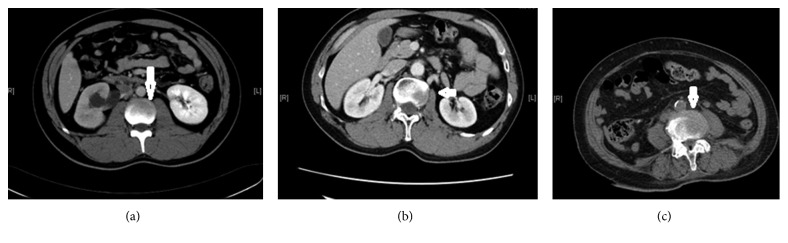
Tumor spread to the vertebral body. CT scans showing tumor spread to (a) the anterior portion, (b) the lateral portion, and (c) the anterolateral portion. Arrows indicate lesions and spreading routes within the vertebral body.
